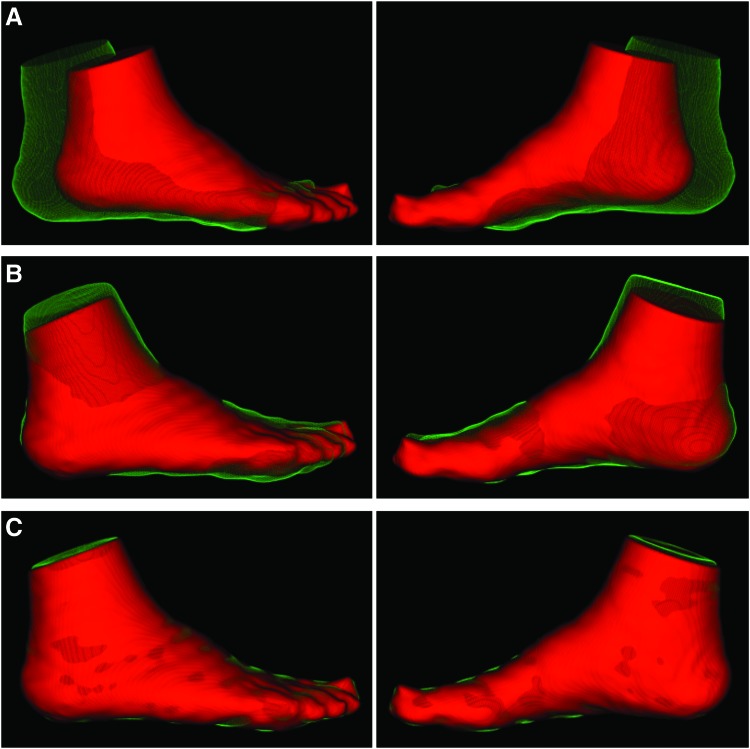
Serial image registration of CT images for evaluating serial changes in angiosome foot perfusion. Lateral and medial views of volume rendered CT images are displayed for the (A) initial foot position at both study time points before image registration, (B) following global rigid registration, and (C) after nonrigid registration. The *red images* represent the foot position at the time of patient's pre-revascularization study visit, while the *green images* represent the change in foot position at the time of patient's follow-up post-revascularization visit. Color images are available online.
